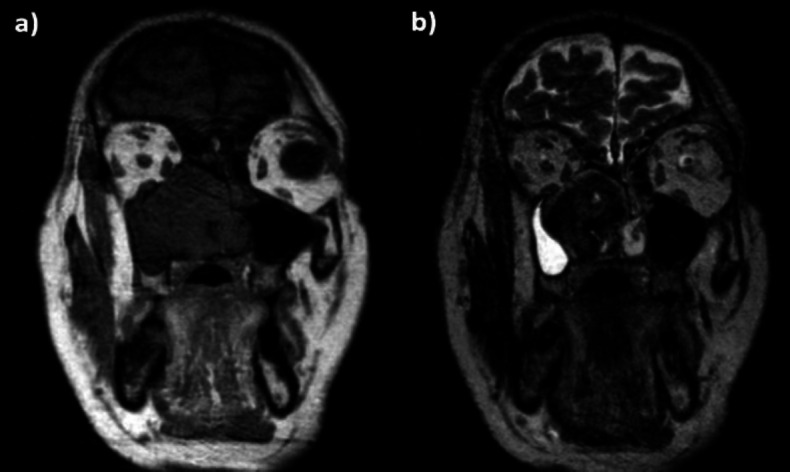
a) T1 weighted MRI, b) T2 weighted MRI
